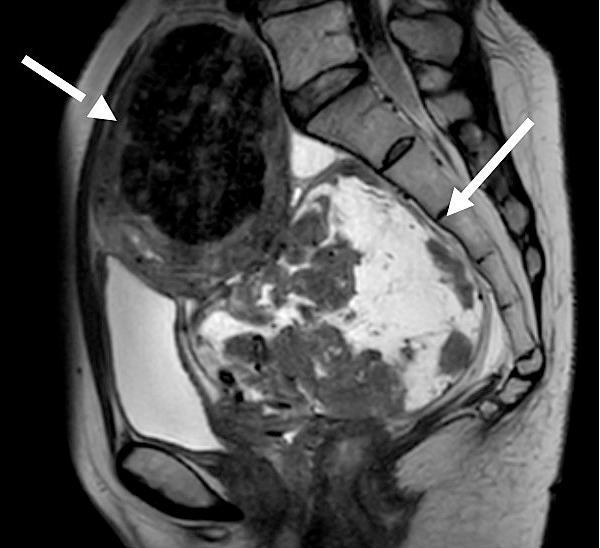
Sagittal magnetic resonance imaging revealed a 58-mm fundal leiomyoma (broken arrow) and a 120 mm × 70 mm retroperitoneal mass (arrow).
